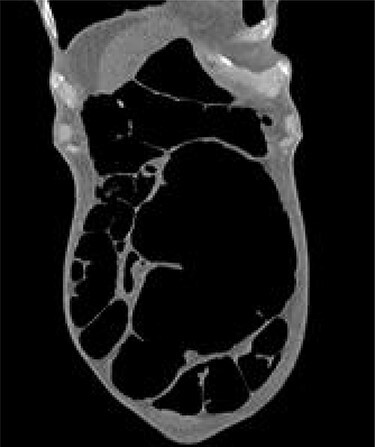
Grossly distended caecum on repeat CT scan.
